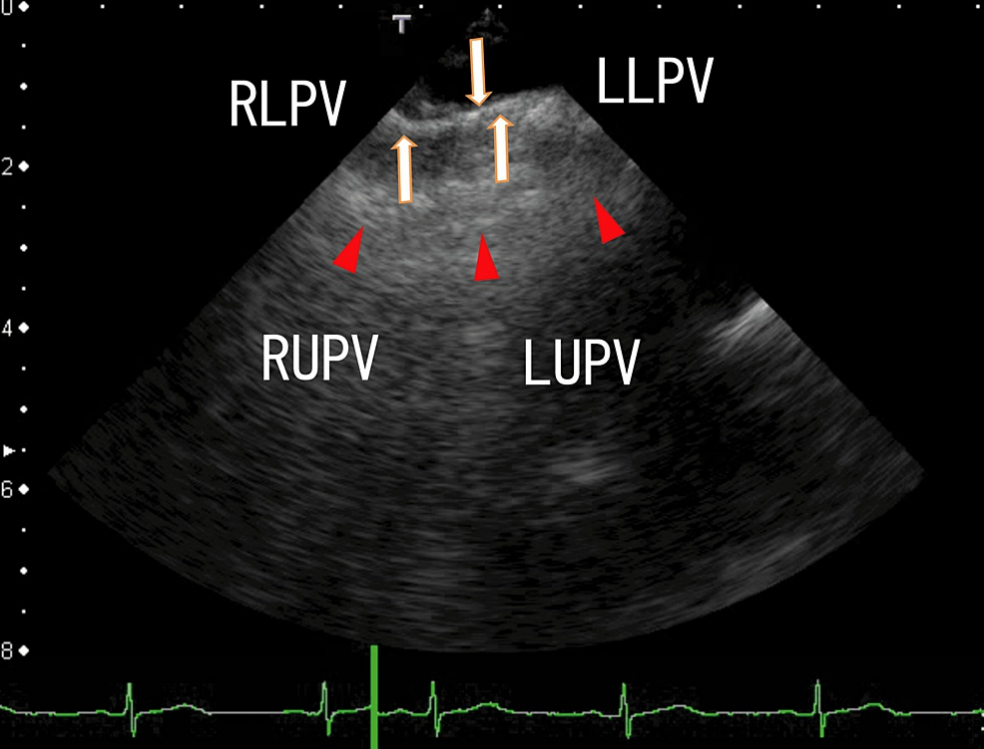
Transesophageal echocardiography (TEE) images showing that the left atrium (LA) thrombi extending from the right lower pulmonary vein (RLPV) thrombi reached the anterior wall of the LA. The arrows indicate the LA thrombi, around which there may be some whitish thrombi. The arrowheads indicate possible walls of the LA. The LA walls had no clear margin; therefore, we hypothesized that there were some thrombi on the LA walls. LLPV, left lower pulmonary vein; LUPV, left upper pulmonary vein; RUPV, right upper pulmonary vein
